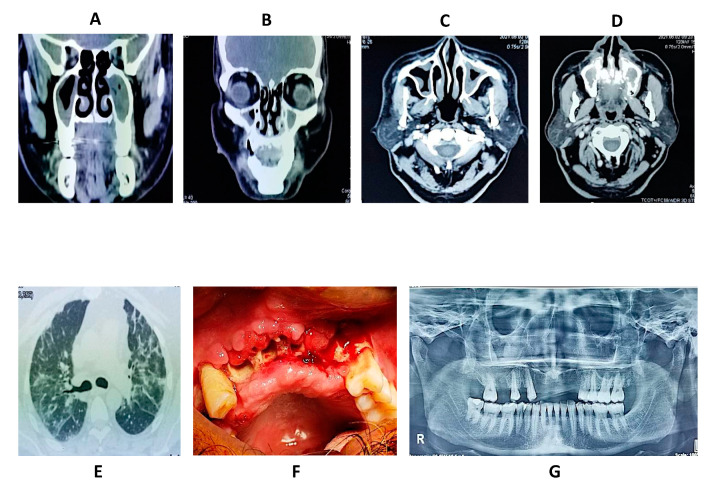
Coronal slices of CT scan with sinus opacification and sequestrum formation (A) and (B). Axial slices of the contrast enhanced CT scan and show involvement of maxillary sinuses and nasal turbinates (C) and (D). An axial slice of HRCT of chest showing post-COVID fibrosis (E). A clinical picture showing non-healing extraction sockets and necrotic bone in the maxilla, the rest of the teeth present were grade I mobile (F). OPG of the same patient showing sinus involvement, thickening of the lamina dura of the extraction sockets in the anterior maxilla (G).
